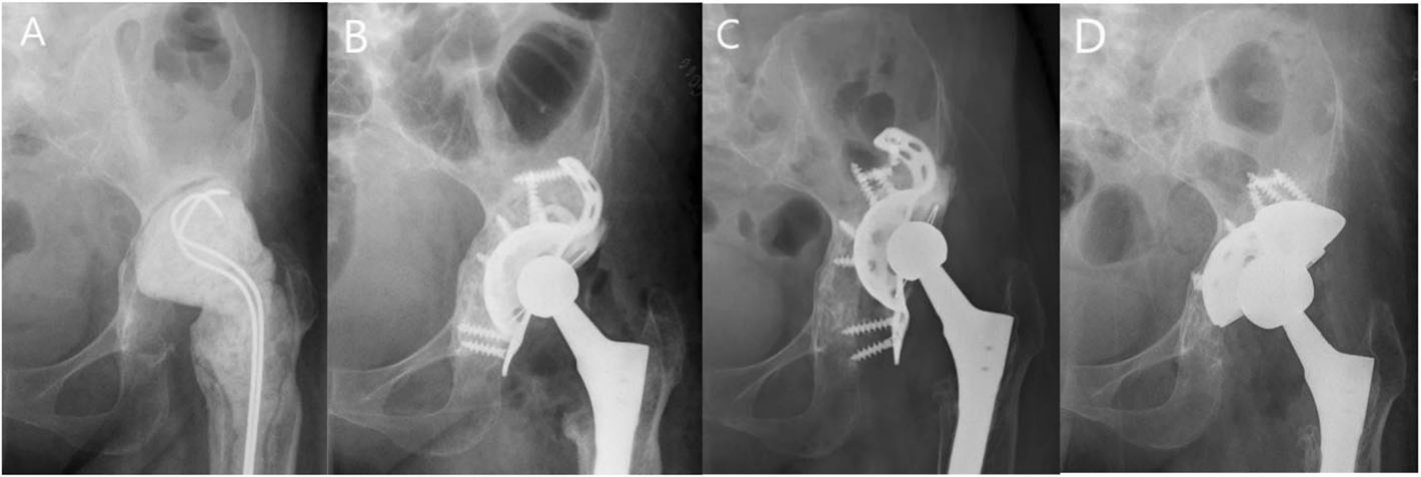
Radiographs of a 51-year-old woman with Paprosky IIIB acetabular bone defects and pelvic discontinuity was found intraoperatively. a Preoperative radiograph. b Immediate postoperative radiograph showed reconstruction cage and morselized allografts reconstructed the bone defect. c Radiograph at 61 months after revision suegery, the cage was loosening with one screw breakage. It was found intraoperatively that allograft was partially incorporated with the host bone and the pelvic discontinuity was healing. d Radiograph after re-revision with jumbo cup and tantalum augment
